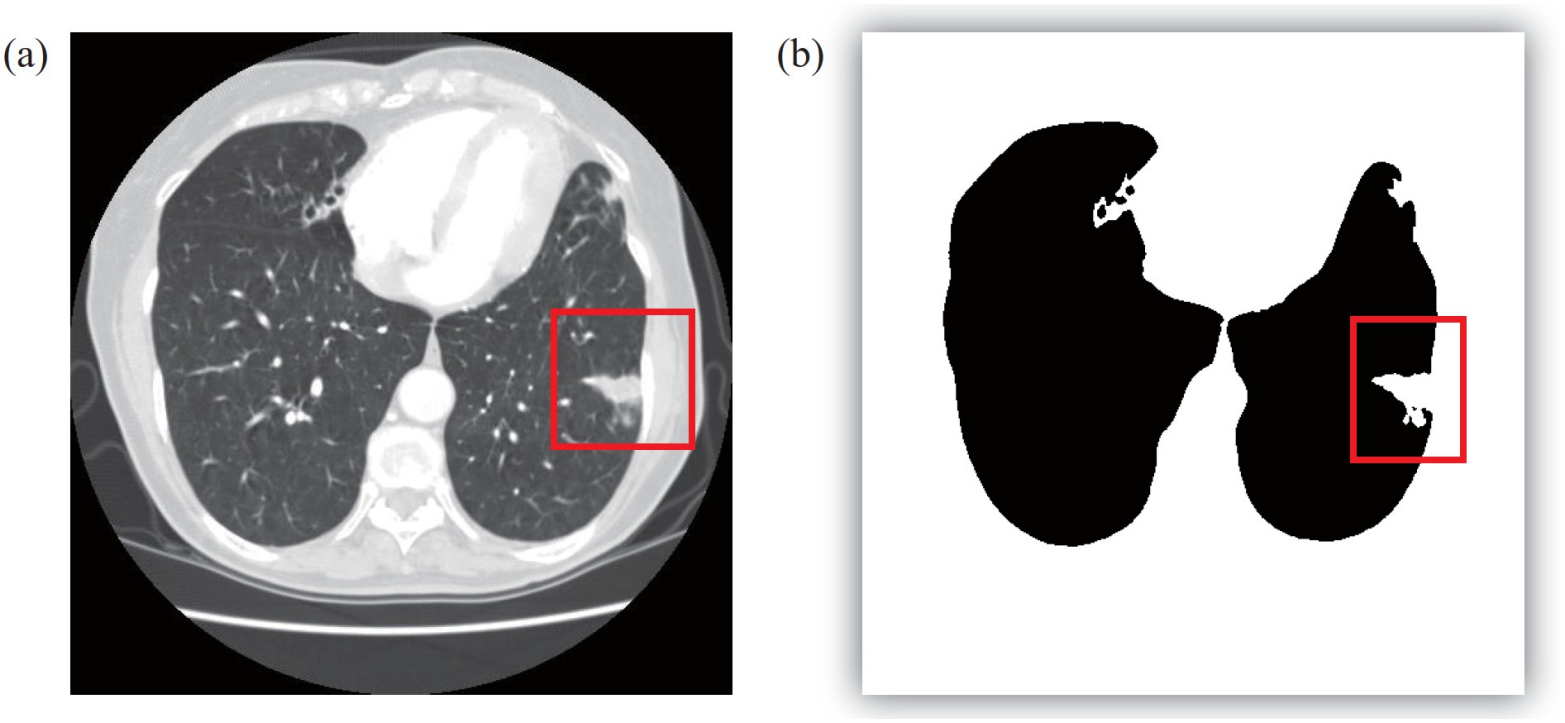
An example of the missed nodal area. (a) An original CT and (b) extracted lung region using a threshold method.
